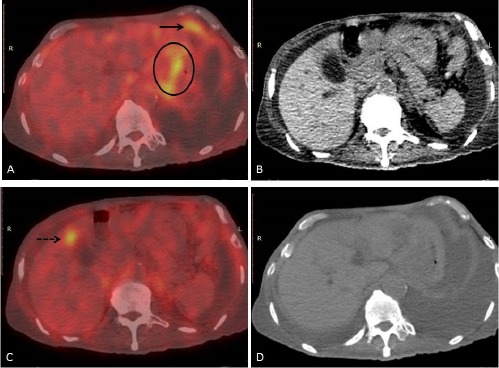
A 67-year-old man with biopsy-proven gastroesophageal adenocarcinoma underwent ^18^F-FDG PET/CT for further assessment. Initial staging CT scan showed a localized primary lesion at the gastroesophageal junction (not shown). However, subsequent PET/CT images showed FDG hypermetabolism at the gastroesophageal junction (circle), peritoneum (arrow), and liver (dotted arrow) (a-d). The management strategy was shifted from curative to palliative. No surgery was performed, and he passed away 3 months after PET/CT
